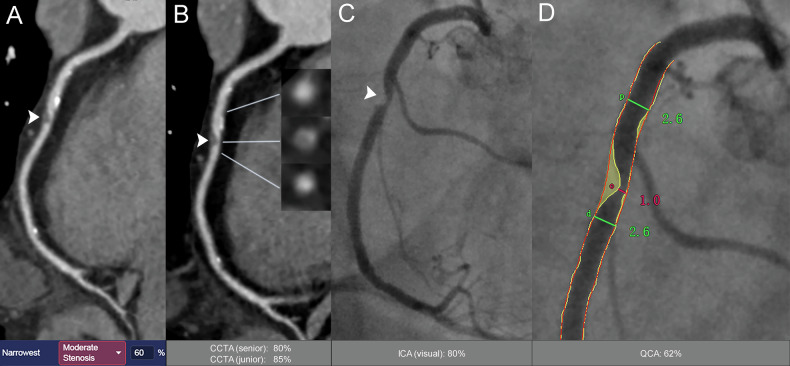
Representative case demonstrating concordance between AI-assisted CT stenosis quantification and QCA. A, B CCTA shows a mixed plaque at middle RCA (white arrowheads). A An AI-reconstructed CPR image. The bottom panel displays the result of AI-aided quantification for the lesion at the middle RCA (white arrowhead), indicating a 60% DS. B Manually reconstructed CPR image. The right panel shows cross-sectional views of RCA from top to bottom: proximal reference lumen, the narrowest luminal site, and distal reference lumen. The bottom panel displays manual CT quantification results: 80% DS by senior radiologists (CCTA (senior)) and 85% by junior radiologists (CCTA (junior)). C ICA shows the lesion at middle RCA (white arrowhead). The bottom panel gives the visual assessment (ICA (visual)) result of 80% DS. D QCA analysis of the same RCA lesion, measuring a proximal reference lumen diameter of 2.6 mm, a minimal lumen diameter of 1.0 mm, and a distal reference lumen diameter of 2.6 mm, yielding a DS of 62% (bottom panel). AI, artificial intelligence; CCTA, coronary computed tomography angiography; CPR, curved planar reformation; DS, diameter stenosis; ICA, invasive coronary angiography; QCA, quantitative coronary angiography; RCA, right coronary artery
